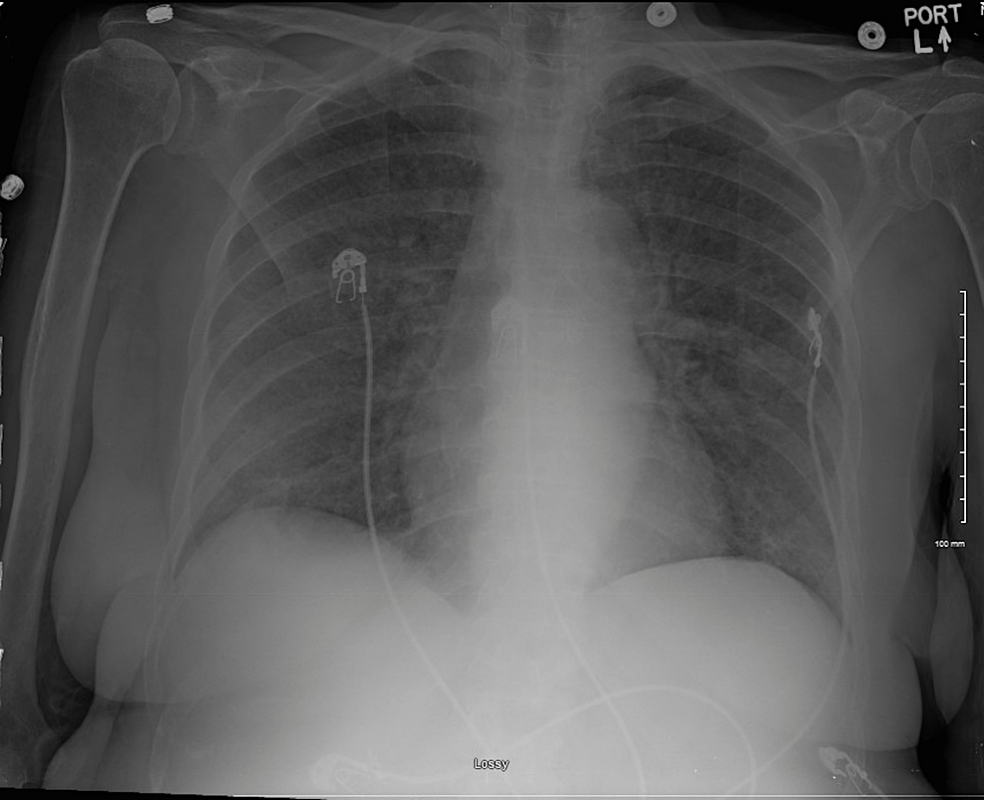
Chest x-ray upon presentation to the emergency department
